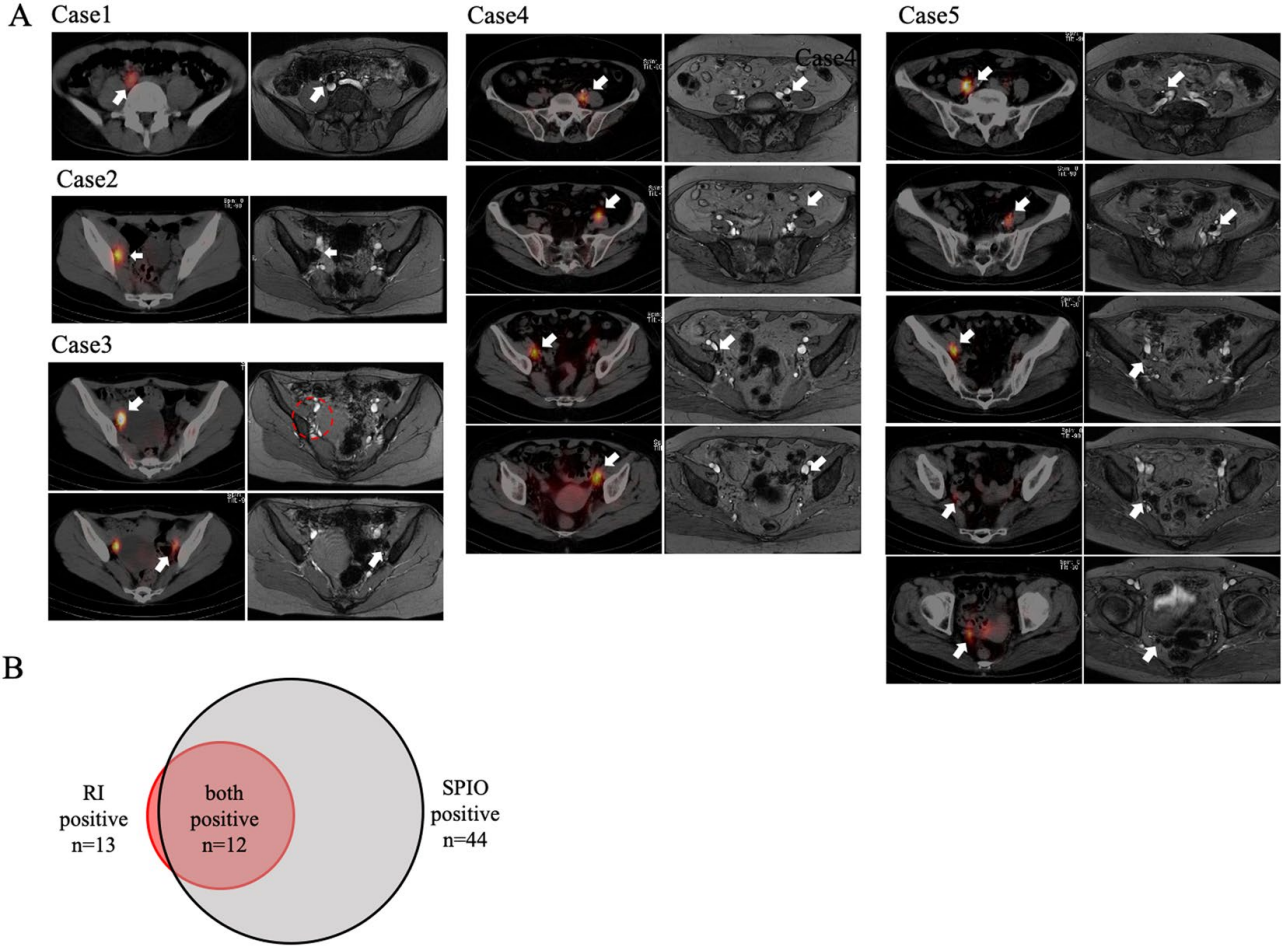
Images from five patients who underwent SPECT/CT and SPIO/MRI. (A) Comparison between SPECT/CT (left) and SPIO/MRI (right). Only the radioisotope-positive LN in Case 3 was not enhanced by SPIO/MRI (red dotted circle). (B) Relationship between radioisotope-positive and SPIO/MRI-positive LNs. Twelve of the 13 radioisotope-positive LNs were also positive for SPIO/MRI. LN: lymph node; MRI: magnetic resonance imaging; SPECT/CT: single-photon emission computed tomography/computed tomography; SPIO: superparamagnetic iron oxide.
